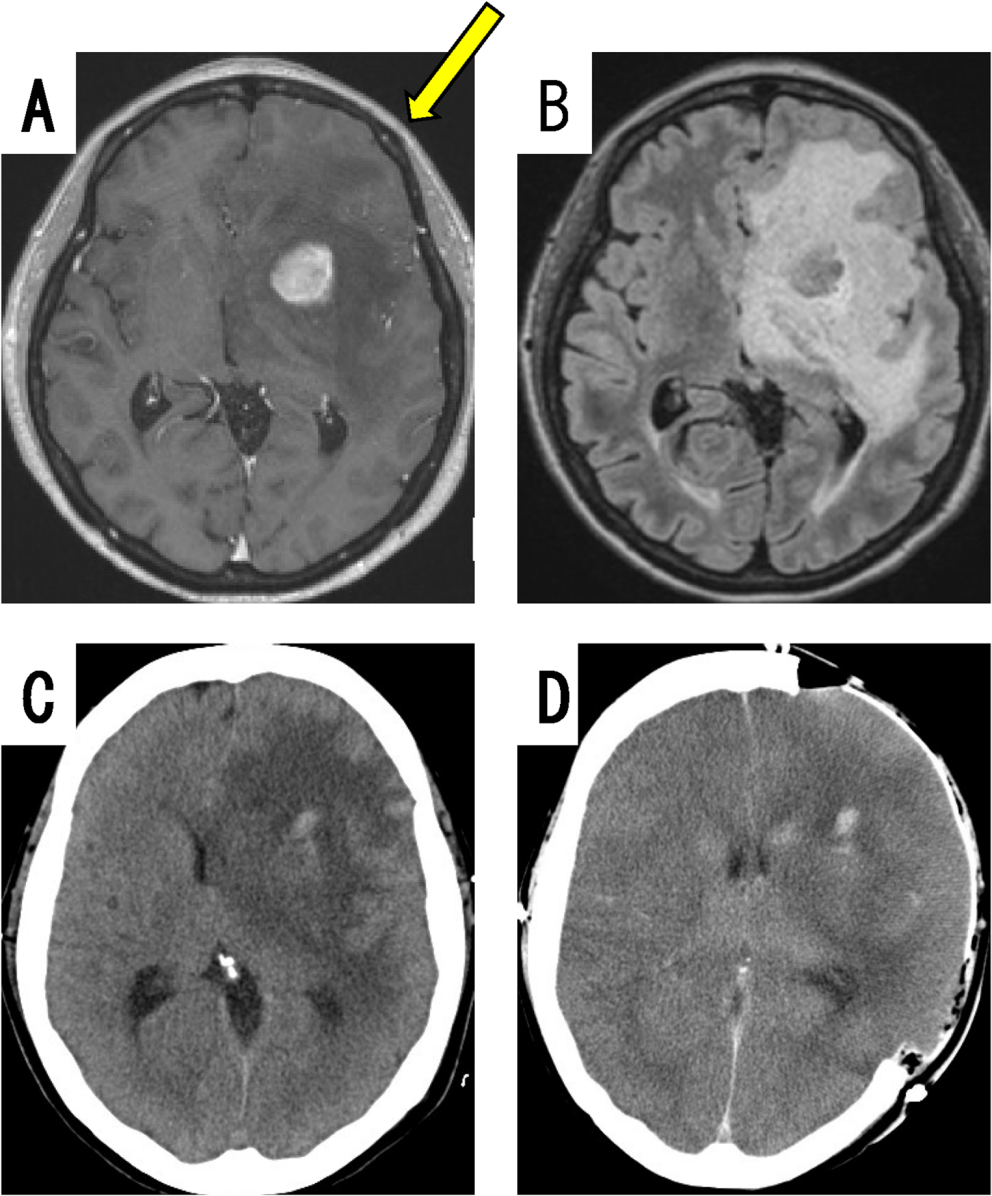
A case of severe brain swelling. Case E: Brain MRI displayed a small enhanced mass in the deep left frontal lobe (A, yellow arrow: direction of sheath insertion), together with severe brain edema in FLAIR (B). Brain CT 27 hours after the biopsy displayed prominent cerebral edema with midline shift (C). Brain CT after external decompression displayed unclear corticomedullary boundaries (D), suggesting severe cerebral dysfunction
